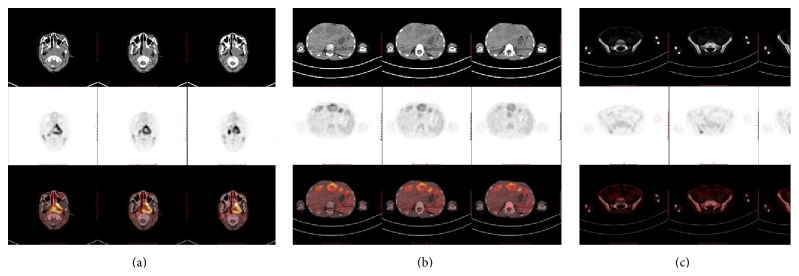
CT (top), PET (middle), and PET/CT (bottom) scans of patient number 3. (a) Intense inhomogeneous ^18^F-FDG uptake in left nasopharyngeal primary mass and asymmetric diffuse soft-tissue thickening in nasopharynx (SUV_max_ 8.2). (b) Multifocal uptake in liver correlating with multiple cystic lesions on CT (SUV_max_ 5.2). (c) ^18^F-FDG uptake in ilium (bone marrow, SUV_max_ 5.0).
